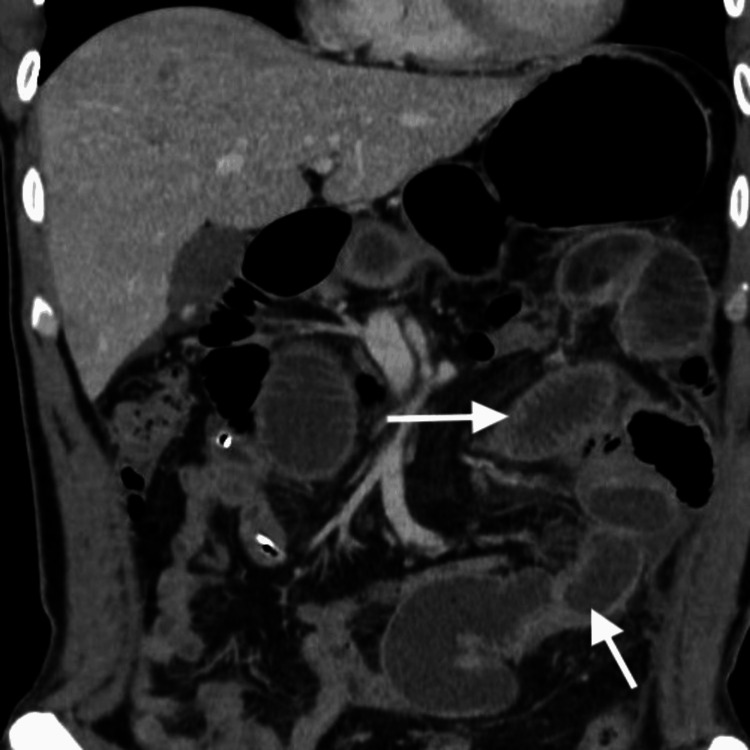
CT scan image of abdomen (Coronal view) Arrow showing postoperative adhesive bowel obstruction with small bowel thickening
